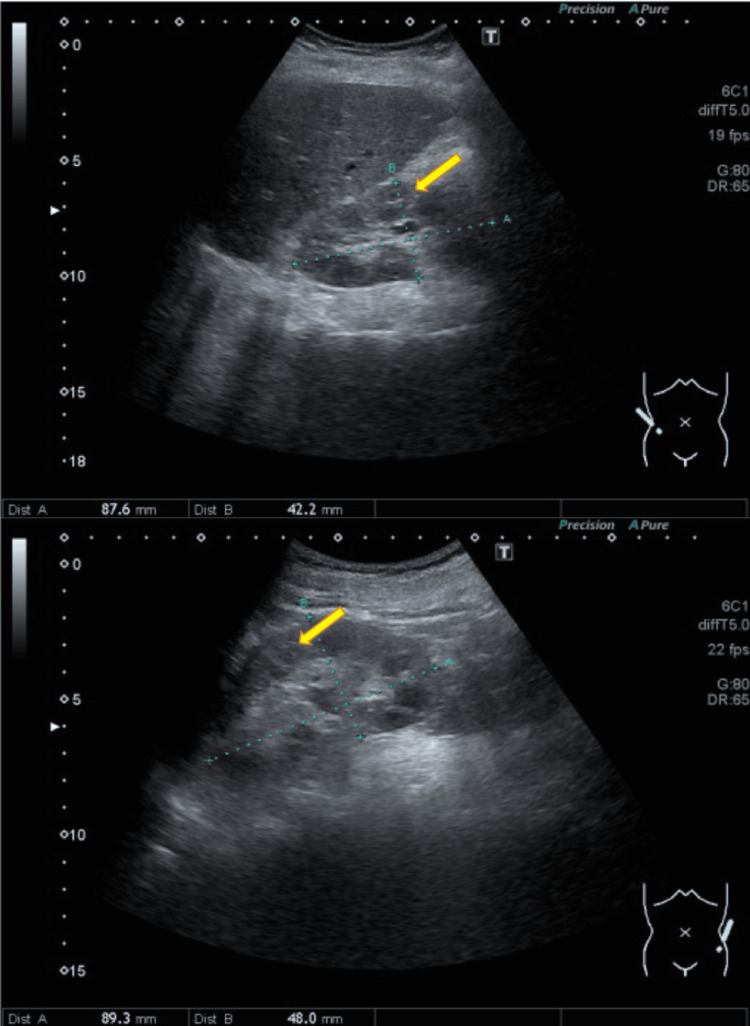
Abdominal ultrasound Here is the abdominal ultrasound examination: The upper panel shows the right kidney, and the lower panel shows the left kidney. There is a slight increase in echogenicity in both renal cortices (indicated by yellow arrows). No abnormalities in blood flow or morphology were detected.
